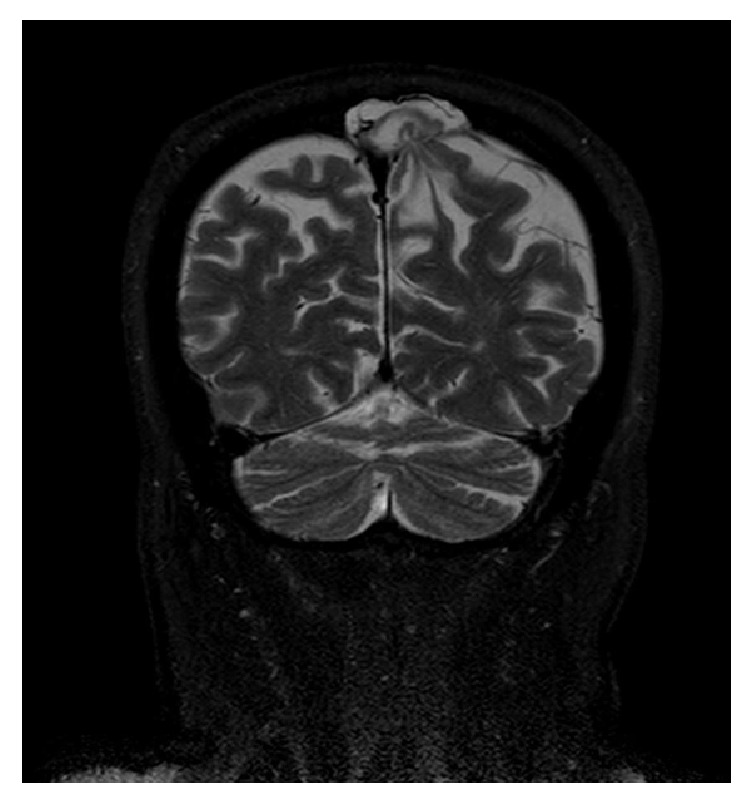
Coronal T2-weighted SPAIR image shows narrow neck and atrophy of the brain herniation.
